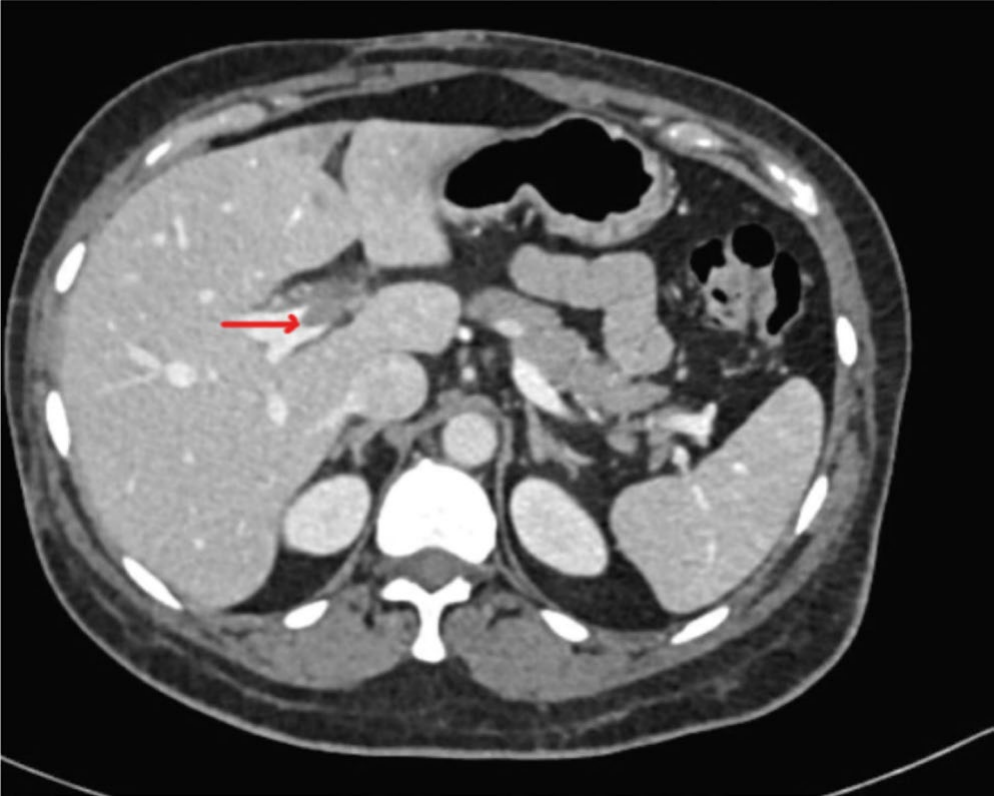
A CT scan of the abdomen with contrast showing portal vein thrombosis.
